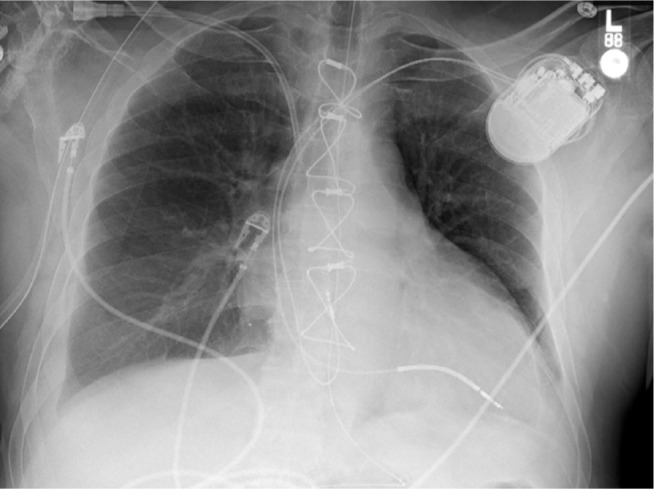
Portable chest X-ray demonstrates standard positions of the atrial lead and single-coil ventricular lead in the right ventricle.
